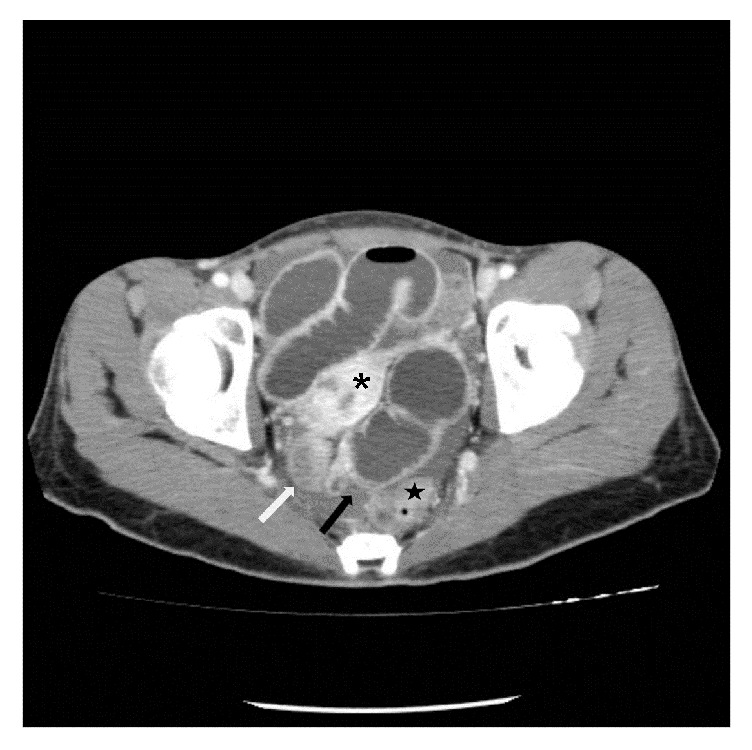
Axial CT image shows distended proximal bowel loop and collapsed distal loop (white arrow) at a transition zone (black arrow) between the uterus (asterisk) and the rectum (star).
